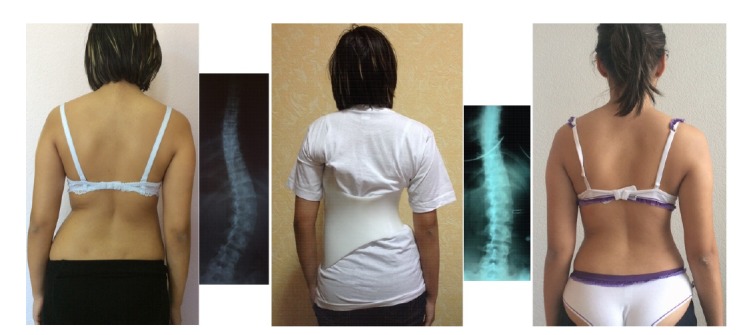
Gensingen Brace^®^ treatment for a single curve pattern of 50 degrees showing a good correction in the x-ray and a significant cosmetic improvement after 6 months of brace wearing time. This Gensingen brace has been made in Ukraine (With kind permission Maksym Borysov, Orttech-Plus Rehabilitation Service, Kharkiv).
